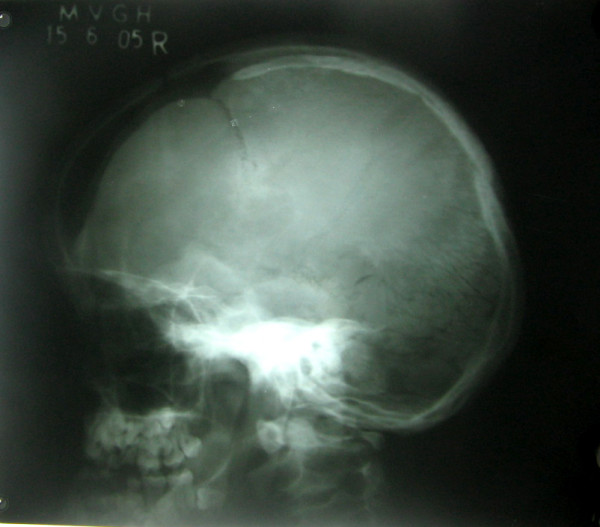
Lateral skull radiograph showing open skull sutures, large fontanels, multiple wormian bones and underdeveloped paranasal sinuses.
